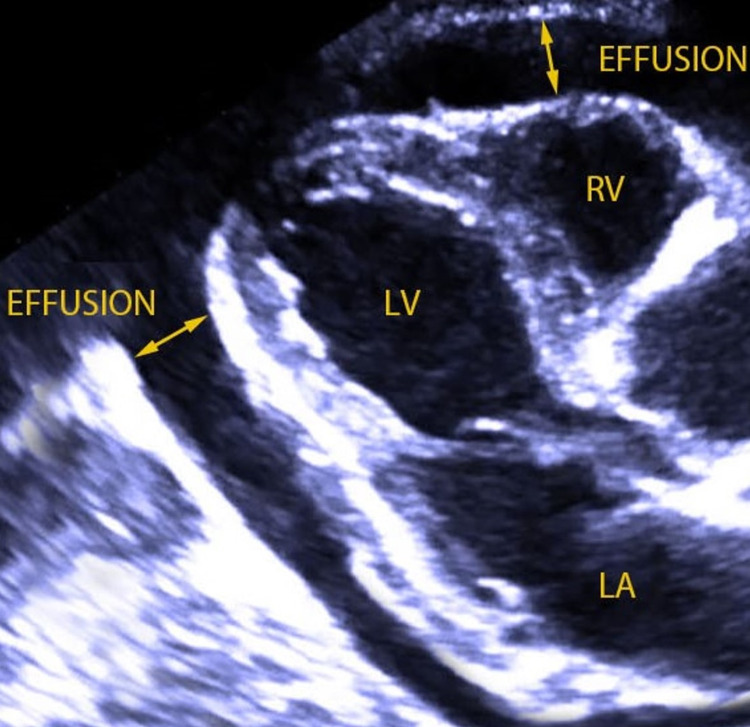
Parasternal long-axis echocardiogrpahy showing pericardial effusion, suggesting pericarditis. LV: left ventricle; LA: left atrium; RV: right ventricle
